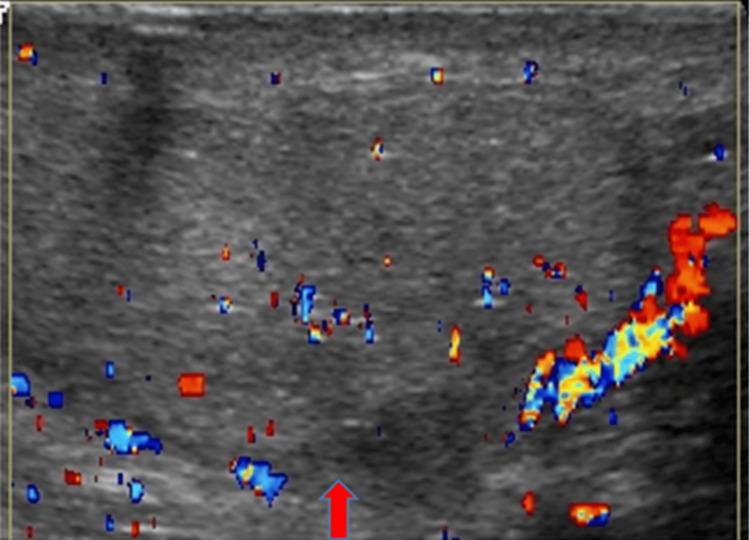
Color Doppler image shows normal flow throughout the testis, except in the area of the fracture (red arrow).
